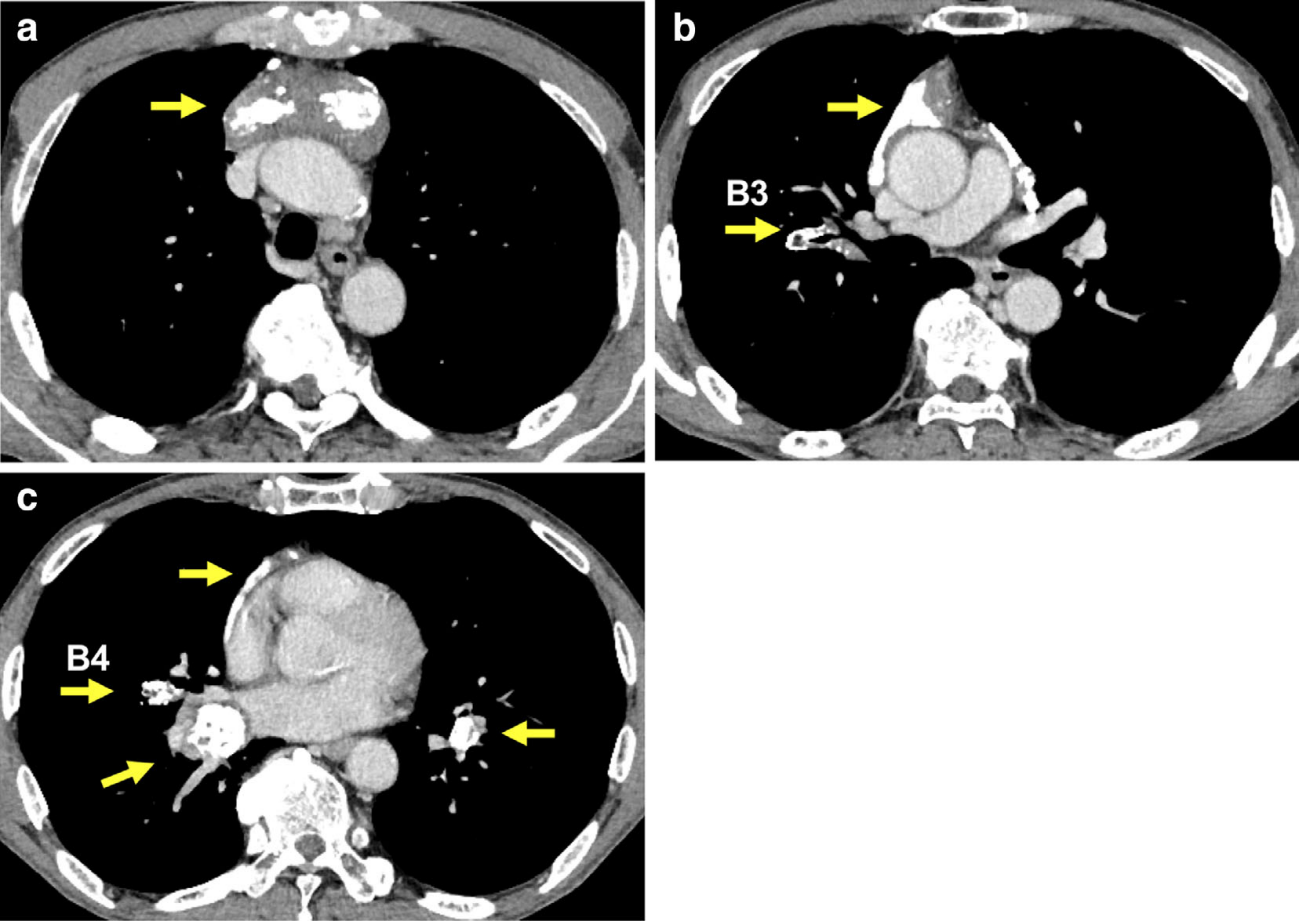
Contrast‐enhanced computed tomography images of the chest. There are markedly calcified tumors (arrows) in the (a) superior anterior mediastinum; (b) surrounding areas of the right B3 bronchus and the prevascular mediastinum; and (c) peribronchial area of the right B4 bronchus and the bilateral lower lobe bronchi.
